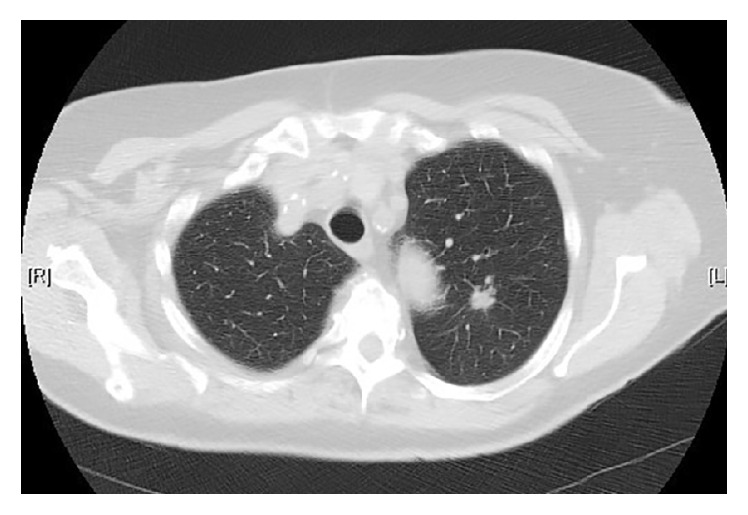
CT of the chest parenchymal view demonstrating a 1.2 cm spiculated left upper lobe nodule.
